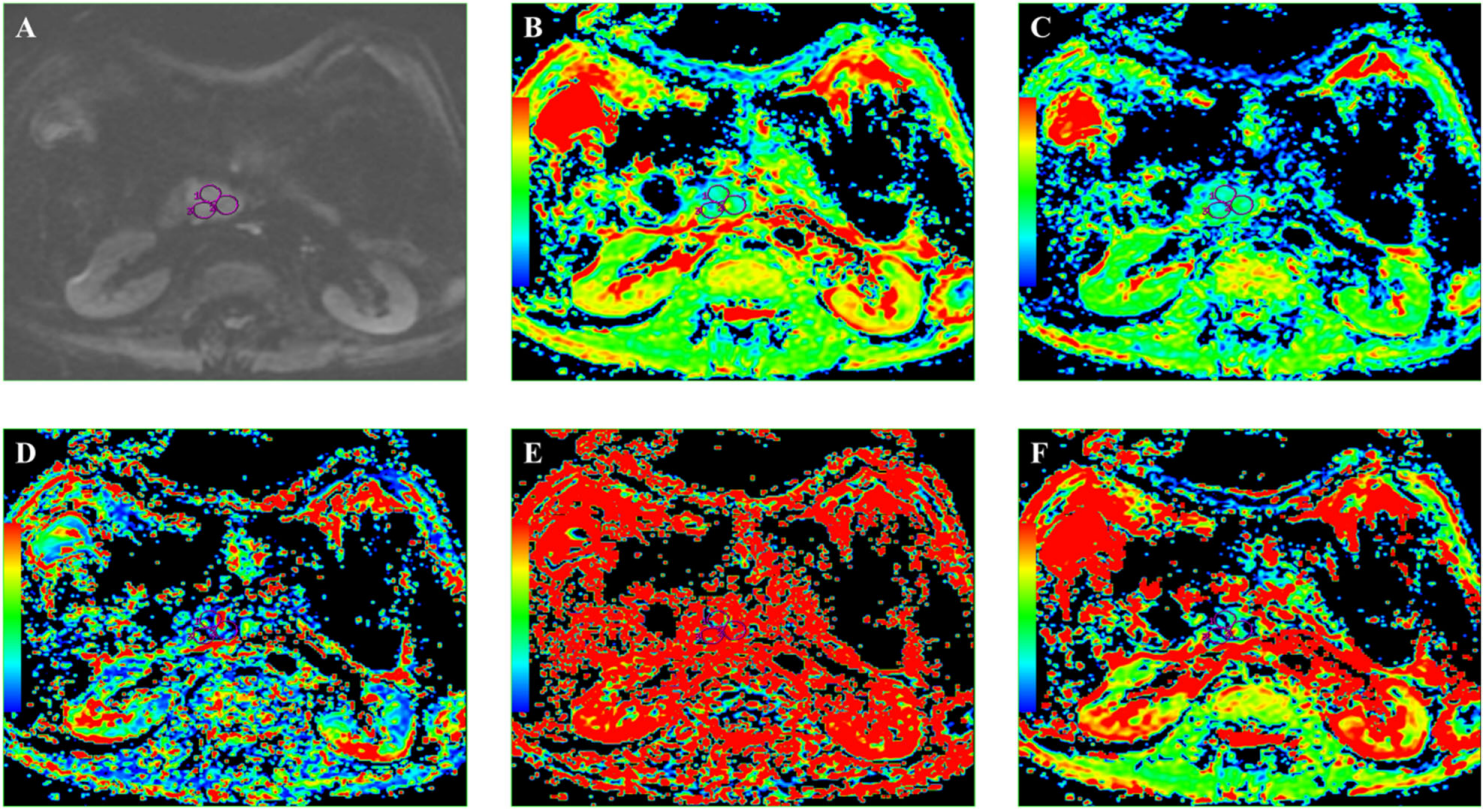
DWI image (A), corresponding ADC map (B), *D* map (C), *D*^*^ map (D), *f* map (E), and DDC map (F) with corresponding ROI measurements
